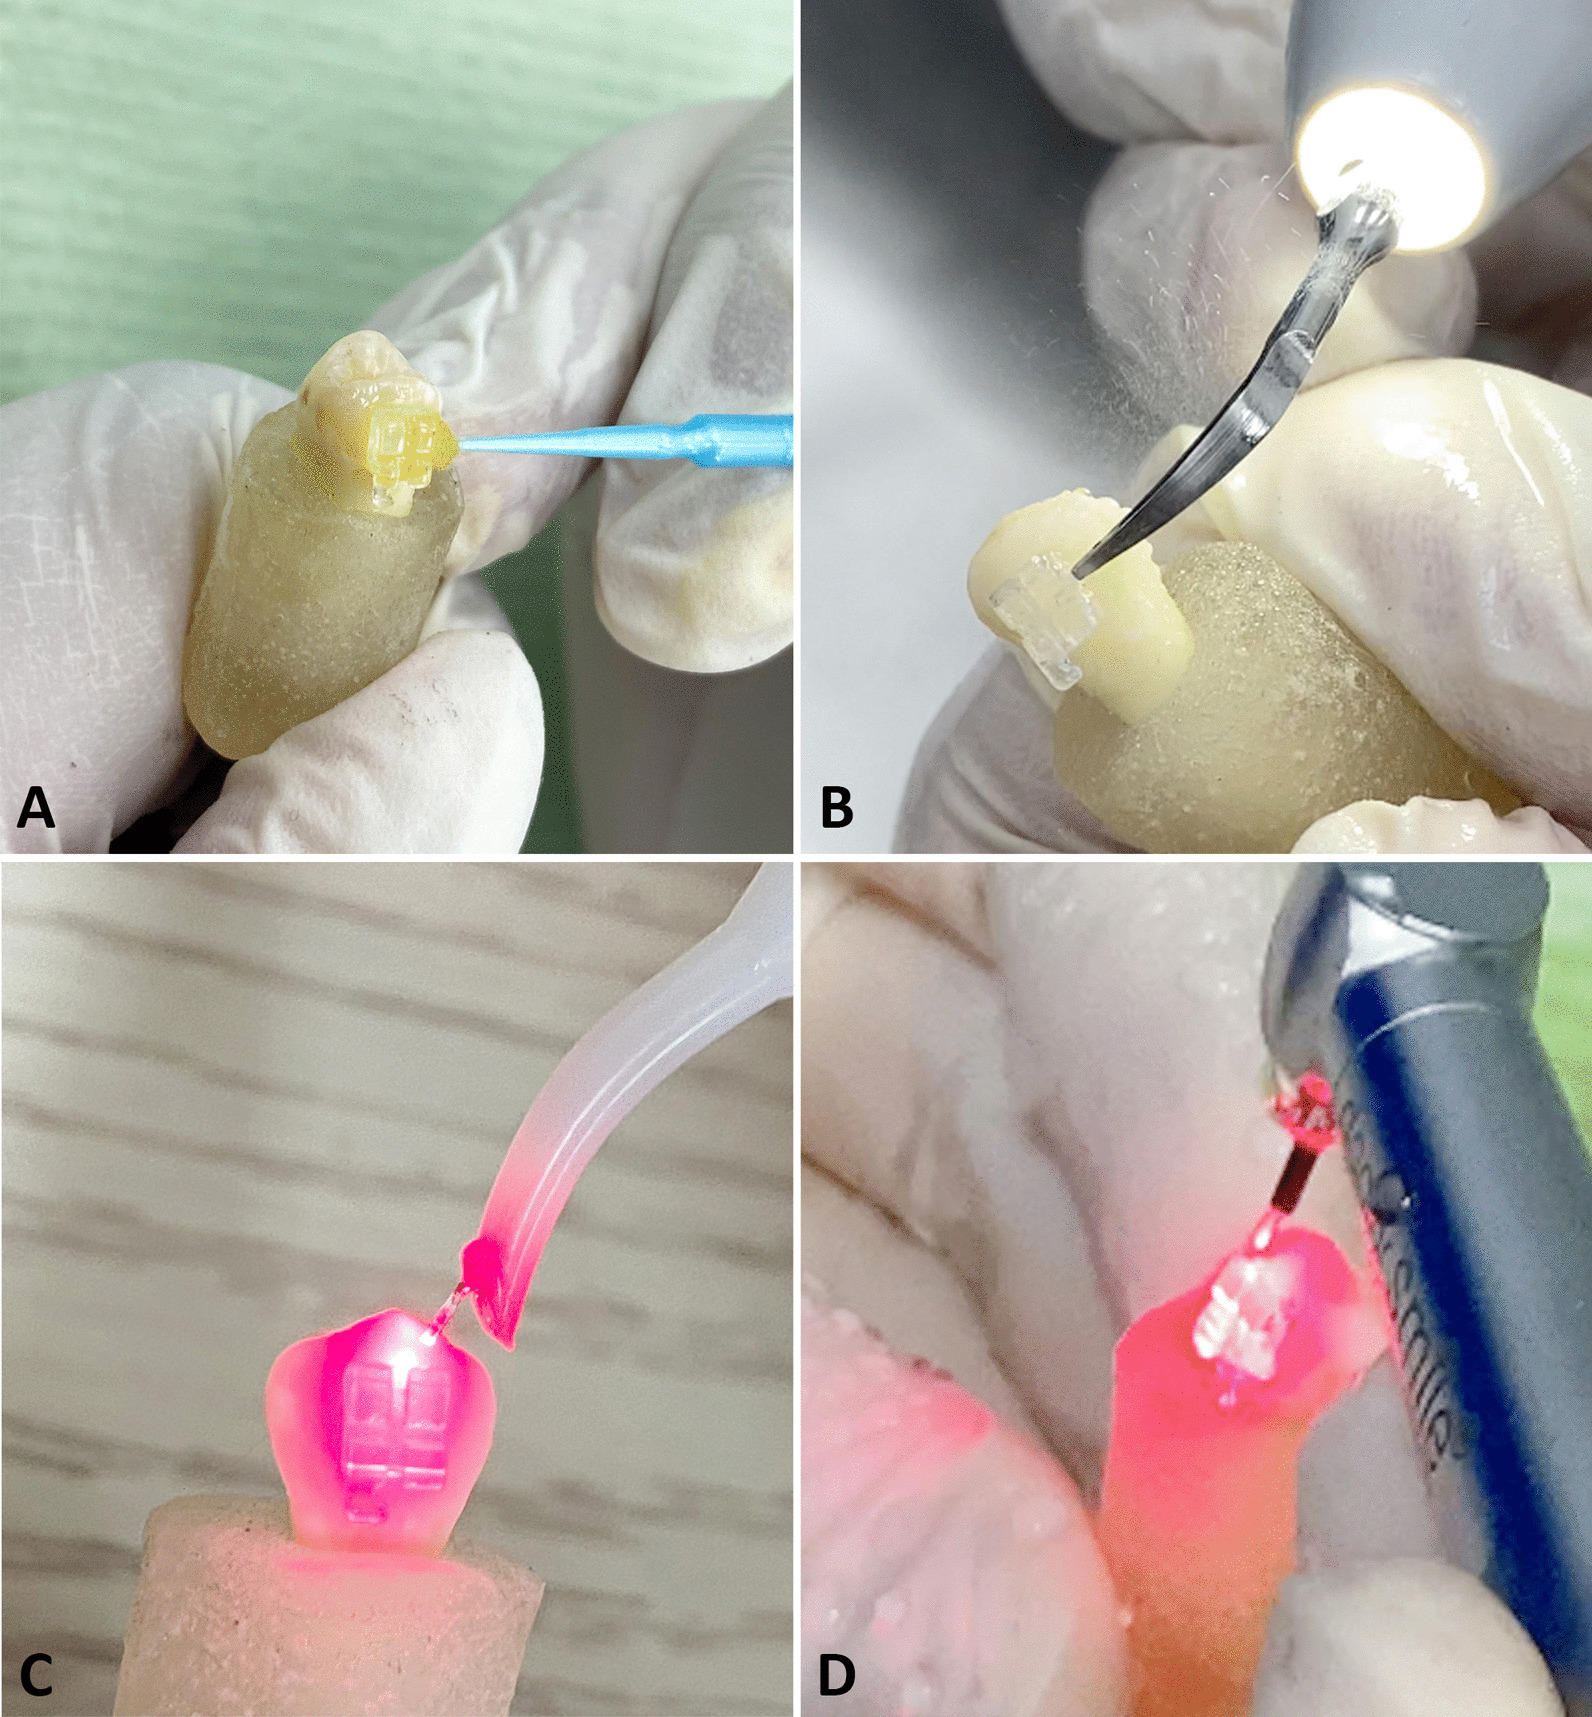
Close-up view of the techniques used A Peppermint oil application, B Ultrasonic application, C Diode laser application, D Er:YAG laser application
